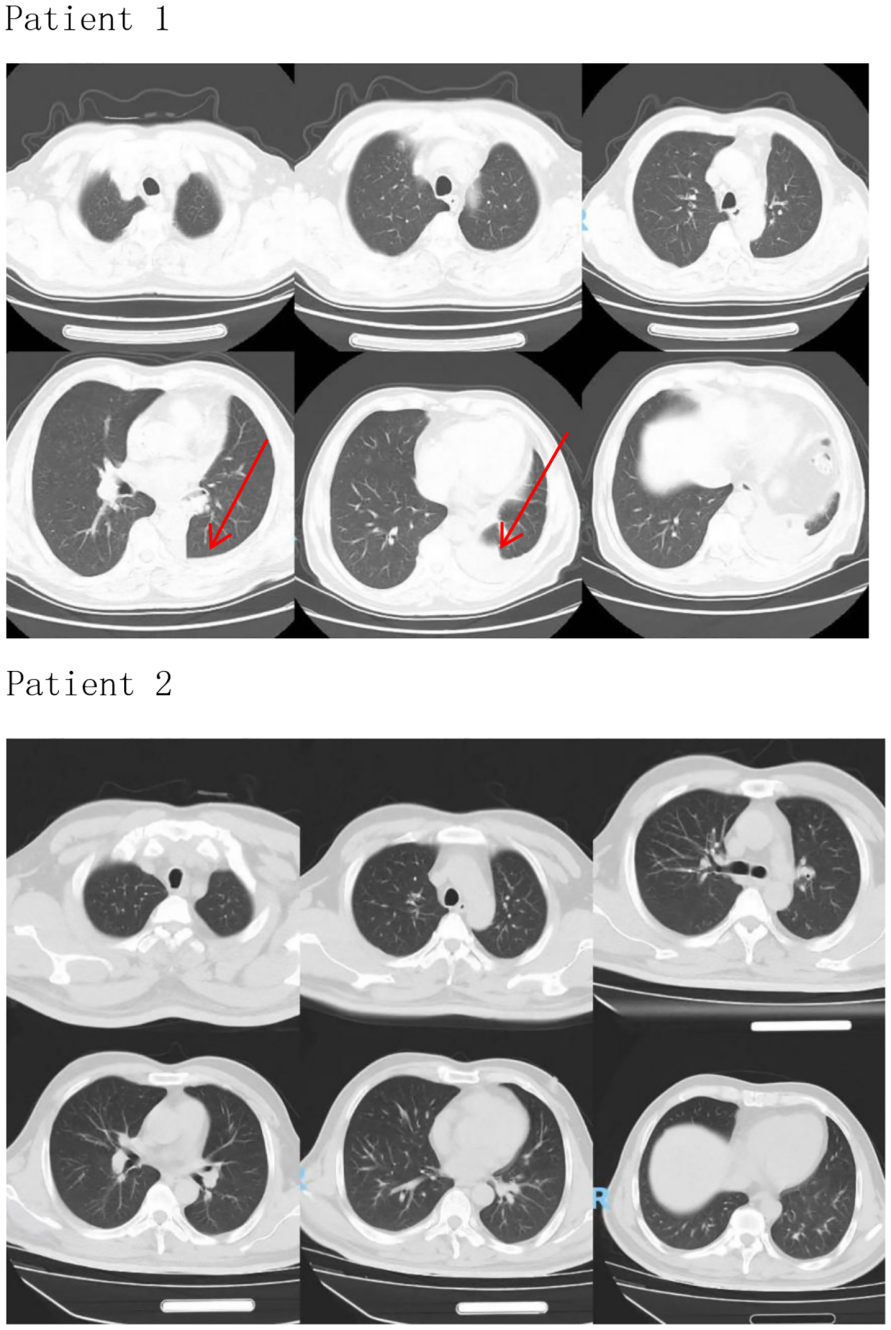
Patient 1 (The arrow indicates the left lower lobectomy site on the CT image.), Patient 2.
